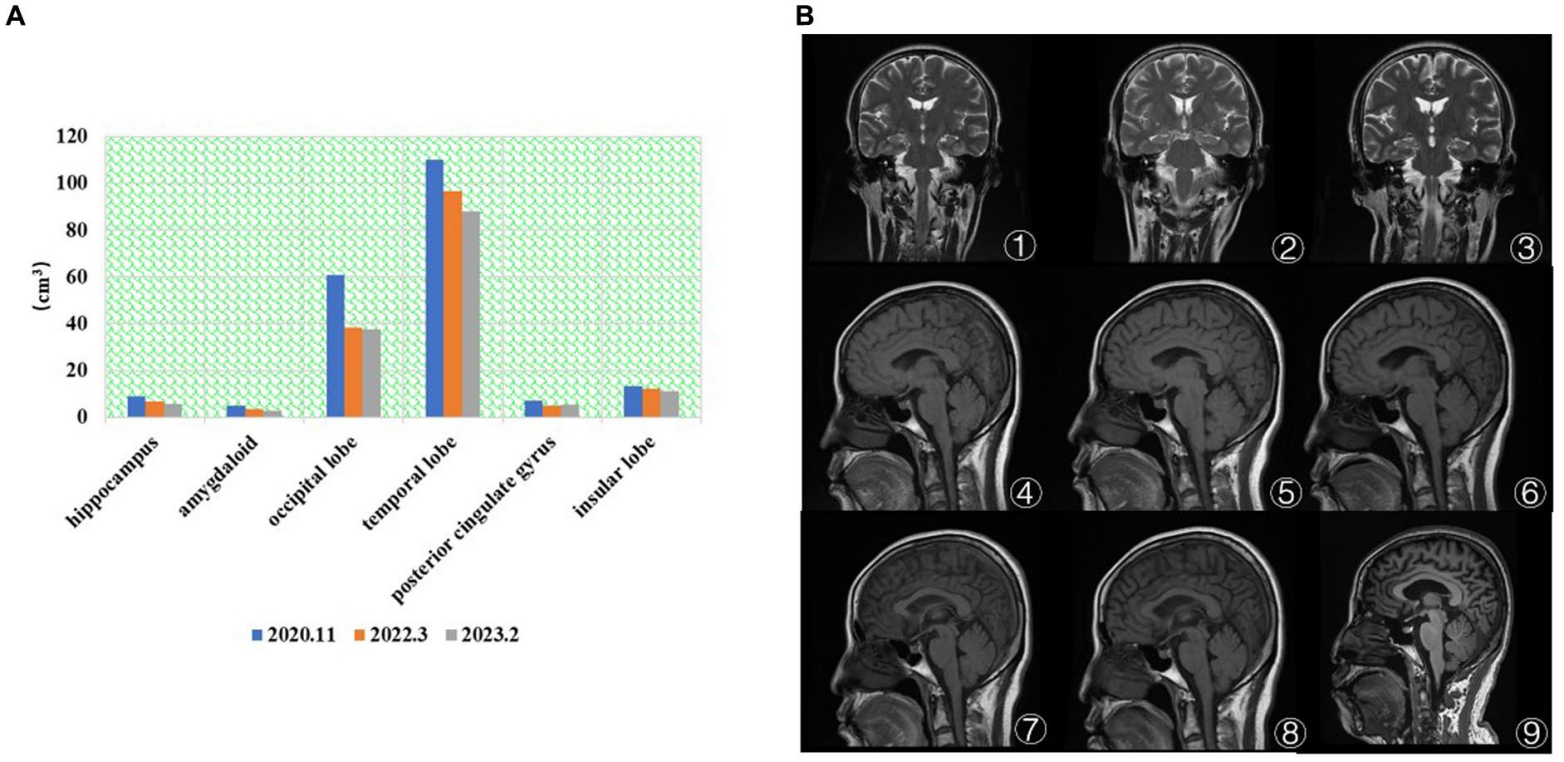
(A) The brain volume changes in regions of interest. The patient’s hippocampal, amygdala, occipital, temporal, and insula brain area volumes have gradually decreased over the last 3 years. (B) MRI results (①–⑨). ① 2014.12 Coronal T2. ② 2017.7 Coronal T2. ③ 2018.3 Coronal T2. ④ 2014.12 Coronal T1. ⑤ 2017.7 Coronal T1. ⑥ 2018.3 Coronal T1. ⑦ 2020.11 Coronal T2. ⑧ 2022.3 Coronal T1. ⑨ 2023.2 Coronal T1. ①–⑥ No significant atrophy in the hippocampal region. ⑦–⑨ The degree of brain atrophy gradually increased.
